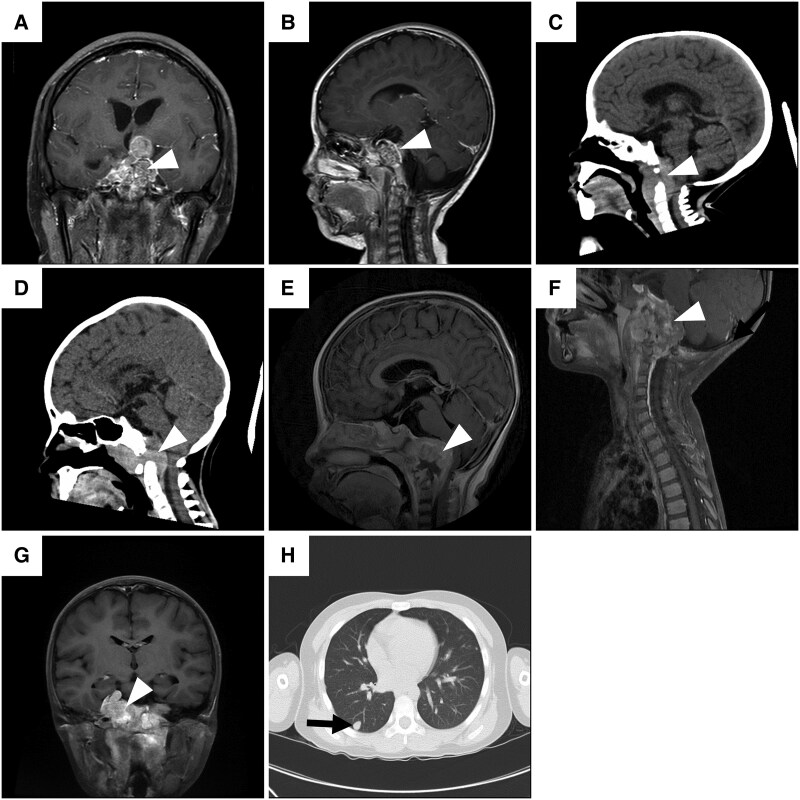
Representative images of paediatric PDCs. Preoperative images of all seven paediatric PDCs are shown. (A) (PDC_1), (B) (PDC_2), (E) (PDC_5), (F) (PDC_6) and (G) (PDC_7): tumours located at the clivus presented an uneven contrast enhancement on the post-contrast T1-weighted image (coronal or sagittal images). In PDC_1, PDC_5 and PDC_7, tumours also involved the sellar and suprasellar region (A), C1- C2 vertebrae (E) and right-side saddle (G), respectively. (C) (PDC_3) and (D) (PDC_4): sagittal CT images show an uneven patchy high-density mass at the clivus with bone resorption and destruction. The tumour sizes were 36 × 51 × 41 mm, 22 × 26 × 39 mm, 42 × 25 × 29 mm, 51 × 48 × 36 mm, 31 × 40 × 45 mm, 49 × 36 × 42 mm, and 58 × 44 × 45 mm for tumours shown in A to G, respectively. (H): PDC_7 with a metastasis in lung. Tumour regions are indicated with white triangles or black arrow. PDC, poorly differentiated chordoma; CT, computed tomography; mm, millimetre.
